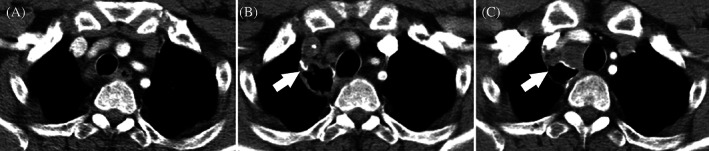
Chest CT images before metastatic esophageal NEC was confirmed. (A) Cancer first diagnosed on November 16, 2018. (B) Assessment after neoadjuvant chemotherapy and esophagectomy on 27 February 2019, and no enlarged lymph node detected. (C) Enlarged 2R lymph node in December 8, 2019.
